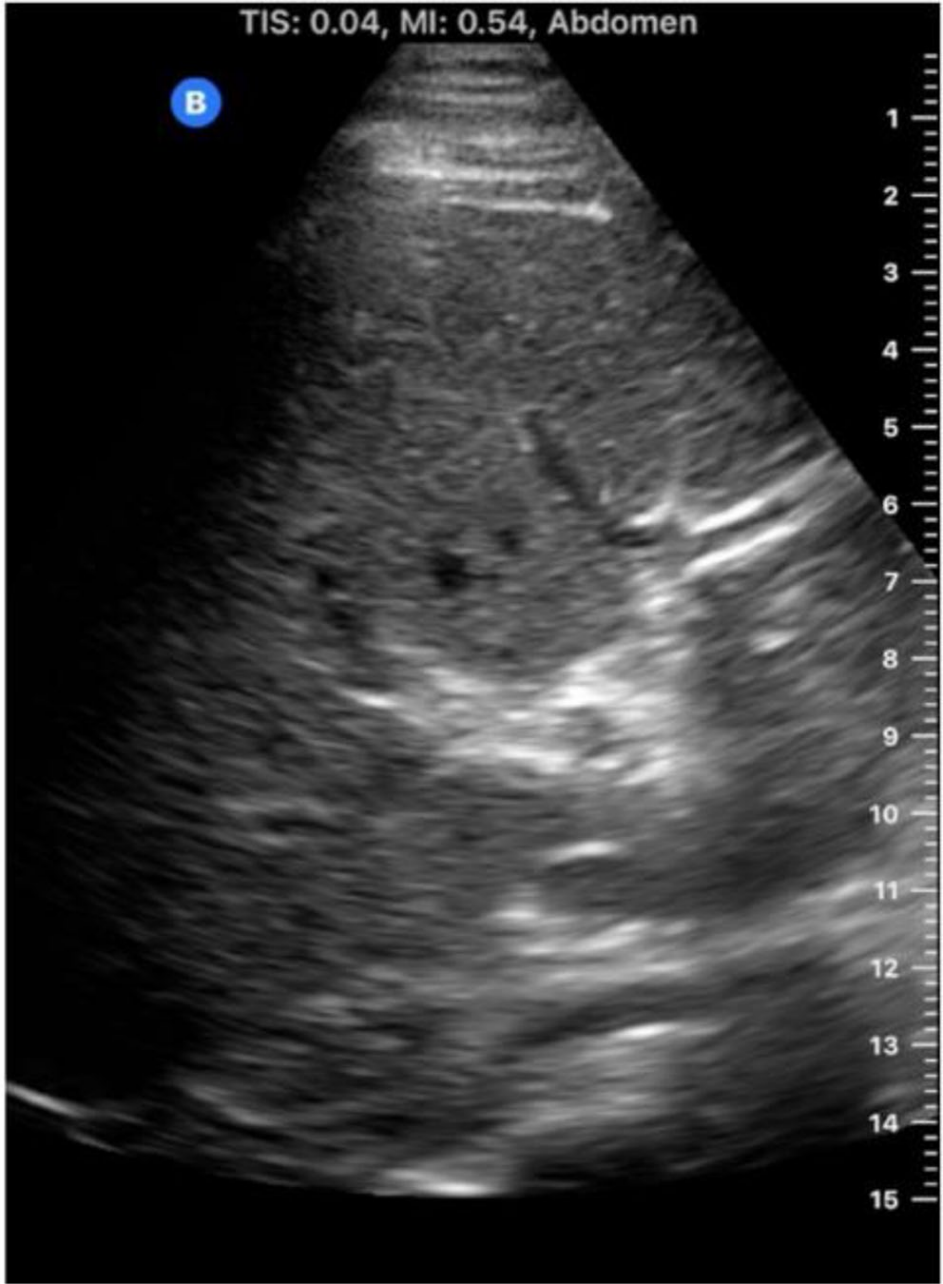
M mode transverse ultrasound of the liver depicting the “jumping deer sign”
